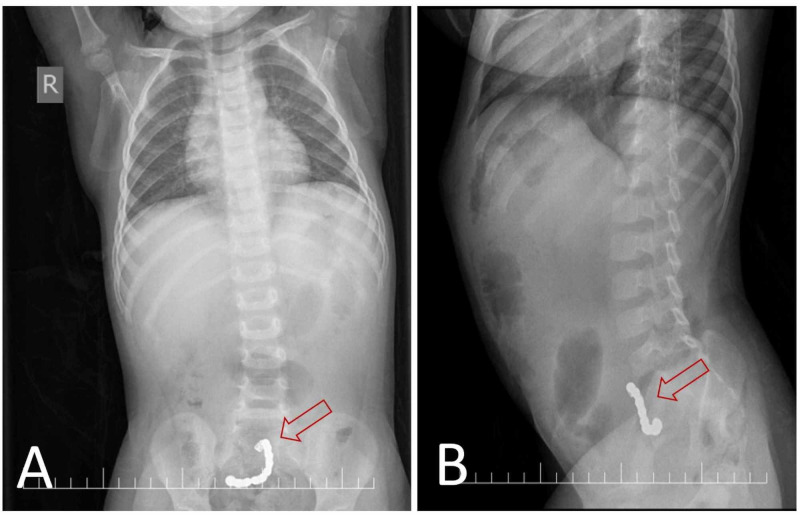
Abdominal X-ray. (A) Postero-anterior abdominal X-ray image. (B) Lateral abdominal X-ray image. Images show a chain of 11 beads extending from the hypogastric region to the right iliac fossa.
